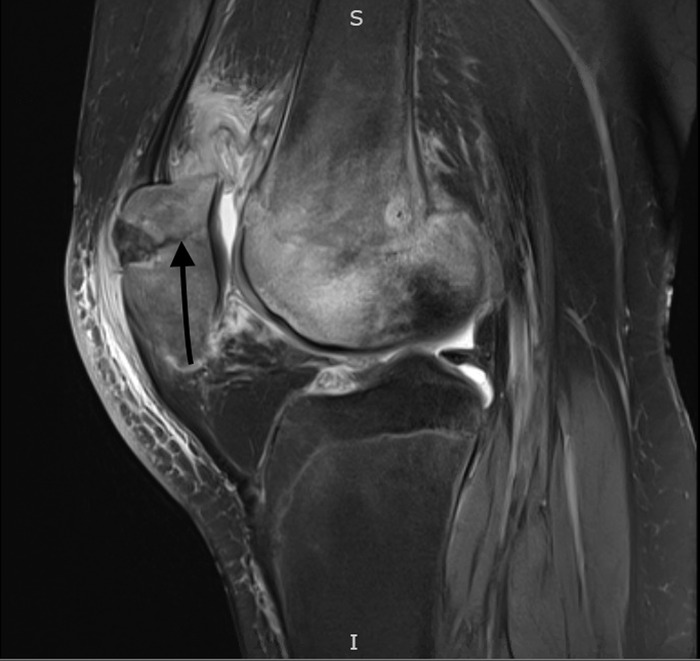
Sagittal fat-suppressed proton density-weighted magnetic resonance imaging shows a left transverse patellar fracture (arrow) after the dislocation occurred at physical therapy.
